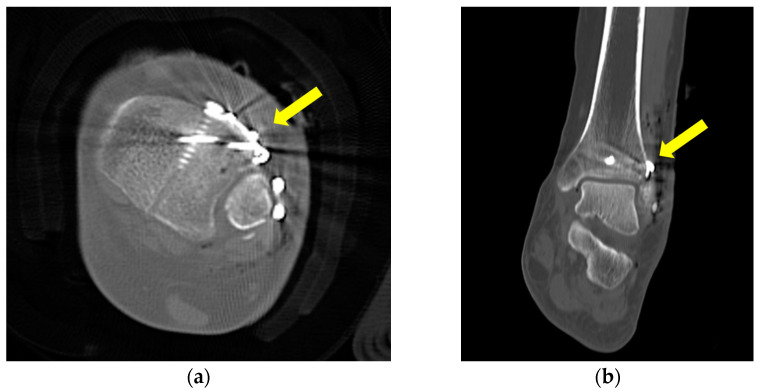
Postoperative axial (a) and coronal (b) computed tomography images demonstrating successful reduction, compression, and fixation of the Chaput fracture, as indicated by the arrow.
